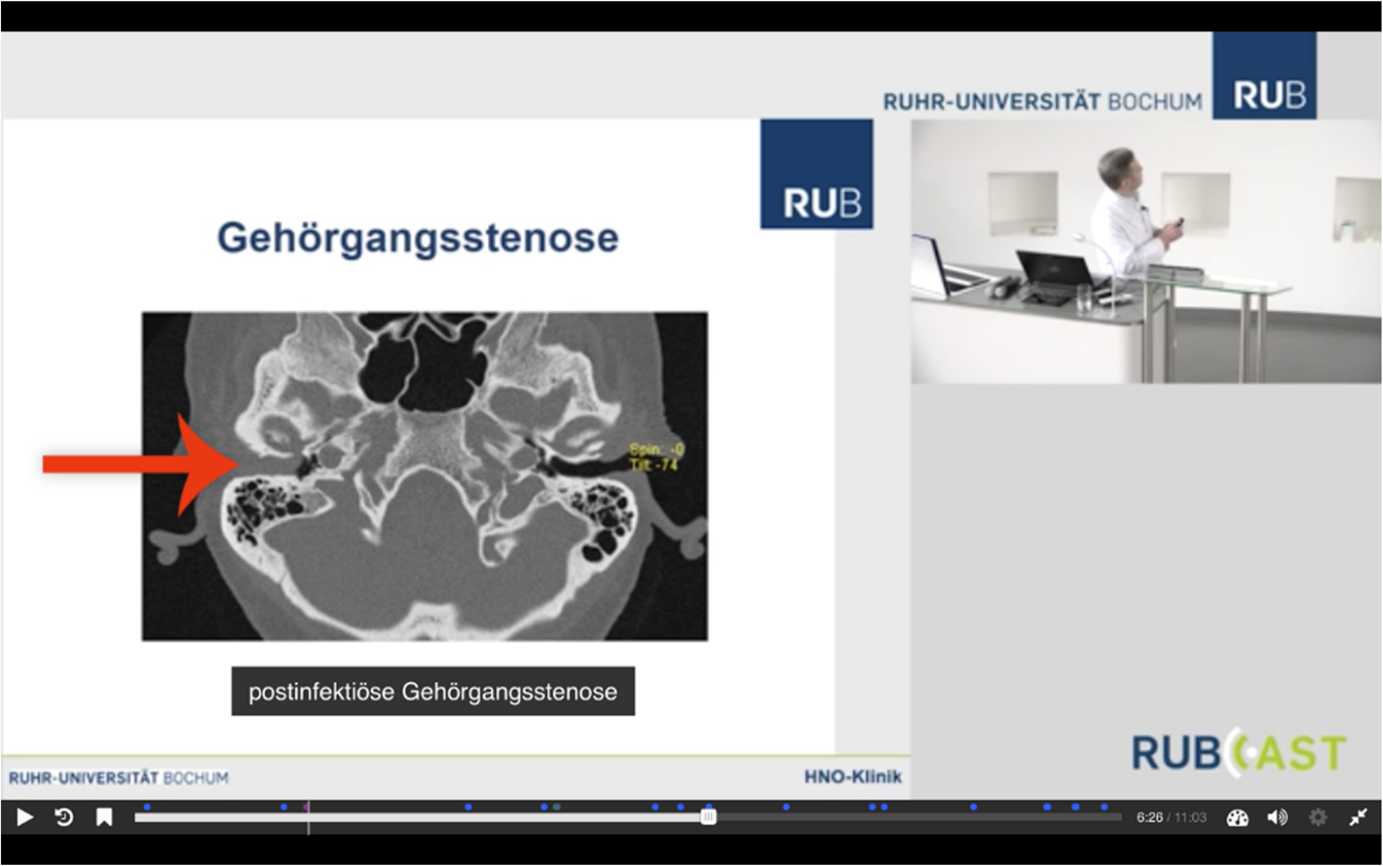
Example for the echo360-compatible design and H5P insert The lecturer is displayed in the upper right corner. To improve quality, slides are not filmed but derived from the presentation file. While pointing on the slide on the wall, the visitor is unable to see the lecturer’s laser pointer. Thus, an arrow was added with the H5P plugin. The caption of the CT scan was also added afterwards with H5P to improve the structure within the video.
